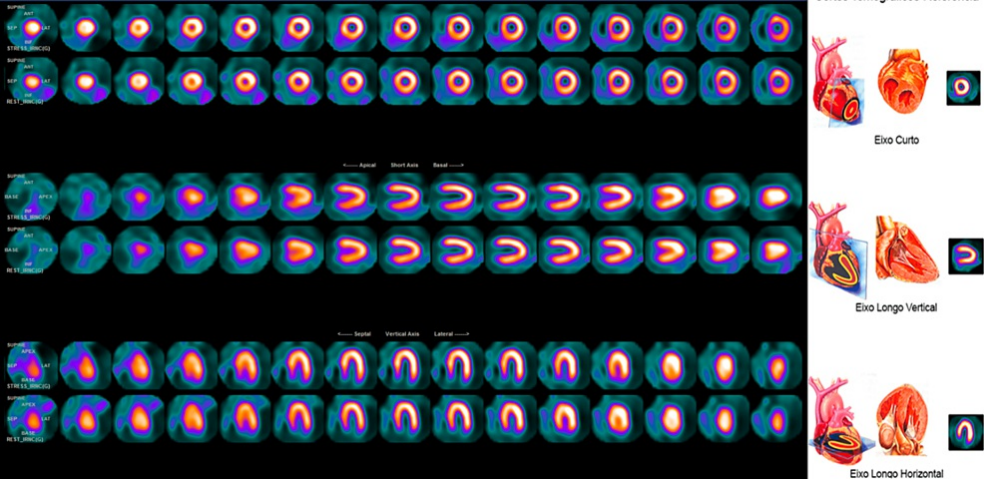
Myocardial scintigraphy showing normal perfusion patterns
